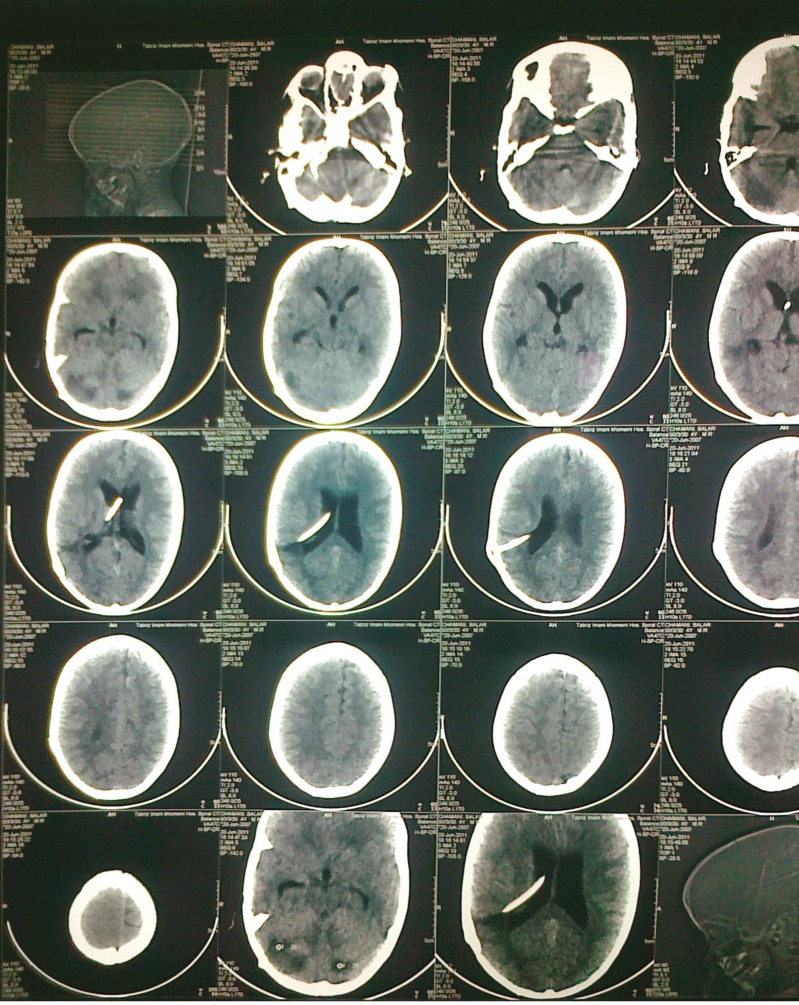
Brain CT scan before extraction of ventriculoperitoneal shunt
